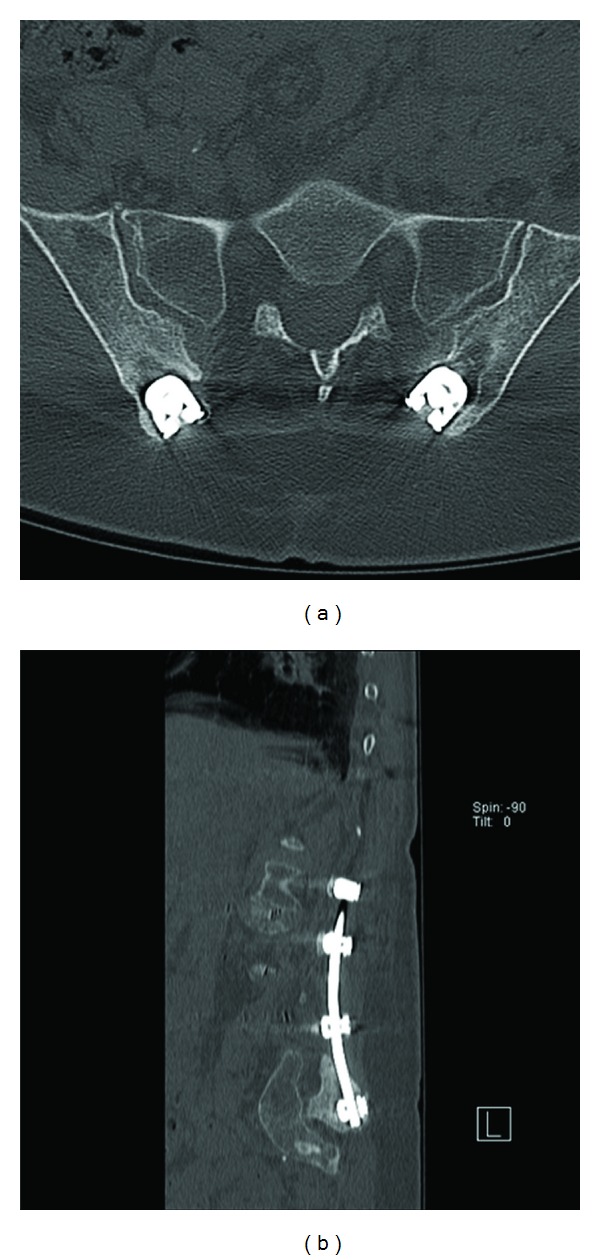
Recession of the iliac screw saddles into the bone to avoid hardware prominence as seen on this postoperative (a) axial and (b) sagittal reconstruction CT scan.
